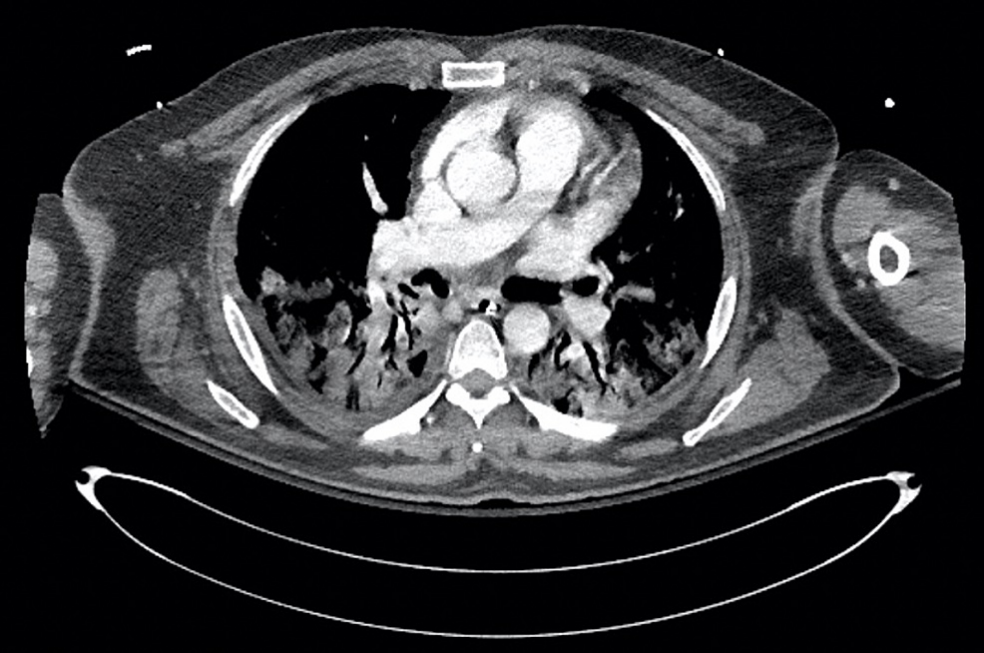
CT chest angiography significant for multiple segmental and subsegmental filling defects in the lower lobe arterial branches as well as extensive bilateral ground-glass and alveolar infiltrates
